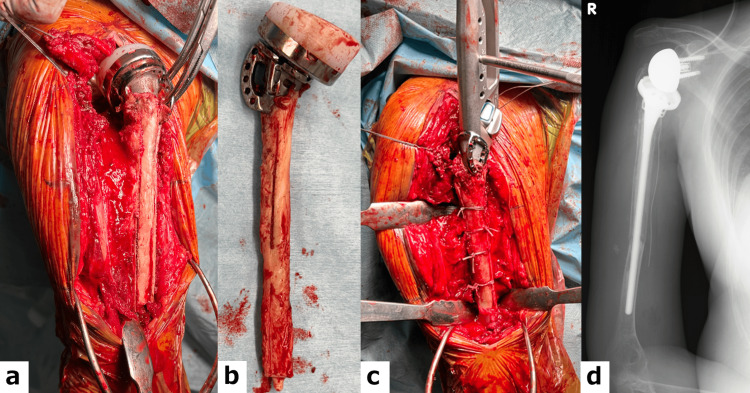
Intraoperative findings and postoperative radiograph (a) The humeral shaft was exposed using the window technique. (b) The removed STD stem showed no signs of osseous integration. (c) The cortical window was stabilized using cerclage wiring, and a long-stem prosthesis was implanted using a cement fixation technique. (d) Postoperative anteroposterior radiograph of the right shoulder showing a long-stem cemented reverse shoulder prosthesis after revision surgery.
